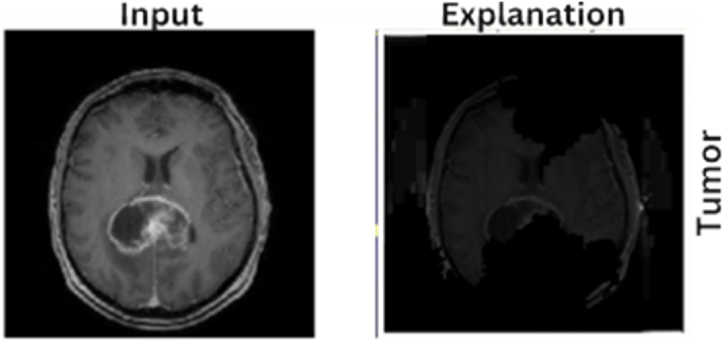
LIME explanation for an MRI image with a tumor, displaying the original MRI (left) and highlighting the presence of the tumor (right).
